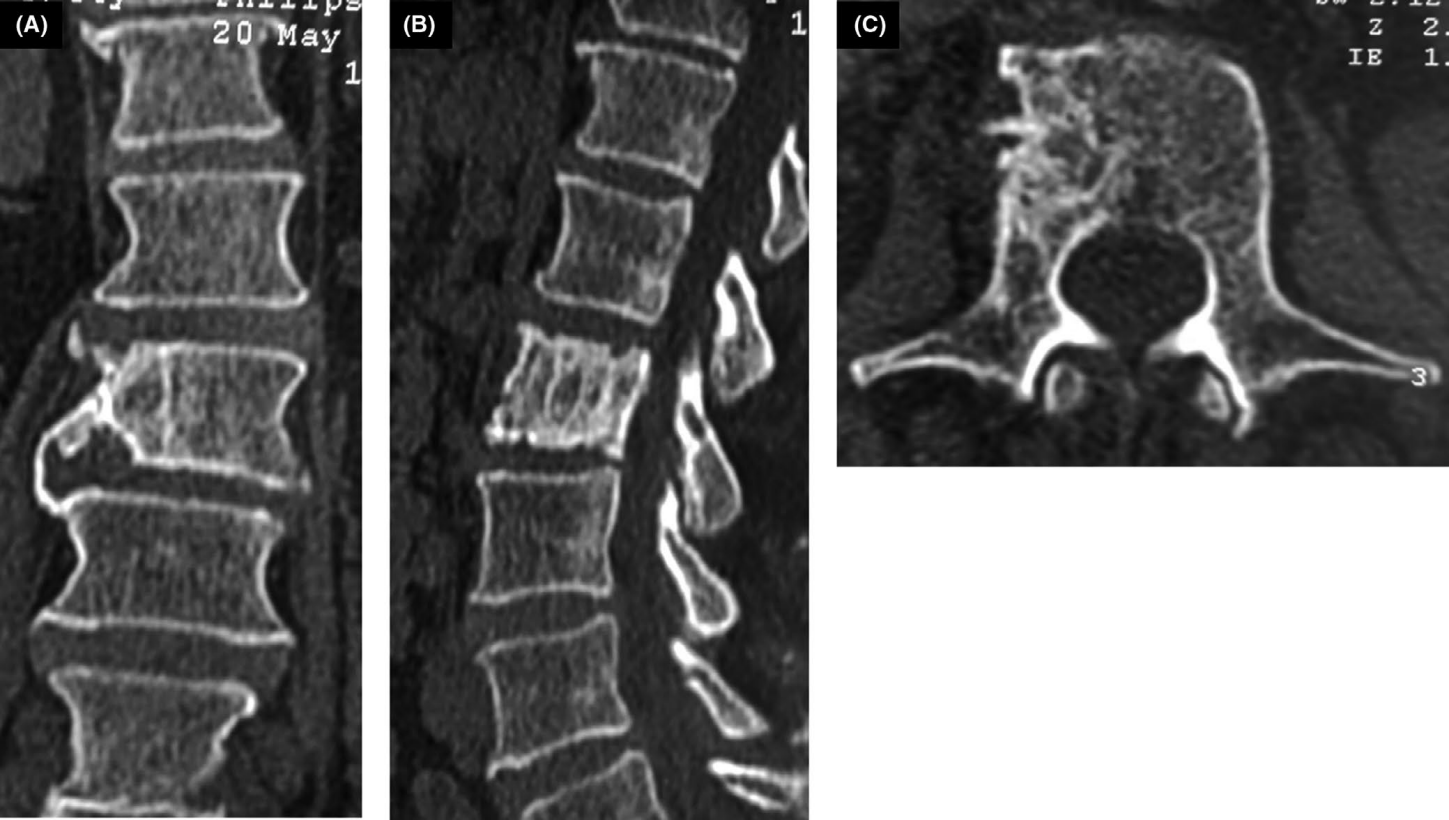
Coronal (A), sagittal (B), and axial (C) CT scans show sclerotic lesion of L2 vertebra with central osteolysis, an extension to the pedicle and cortical destruction
